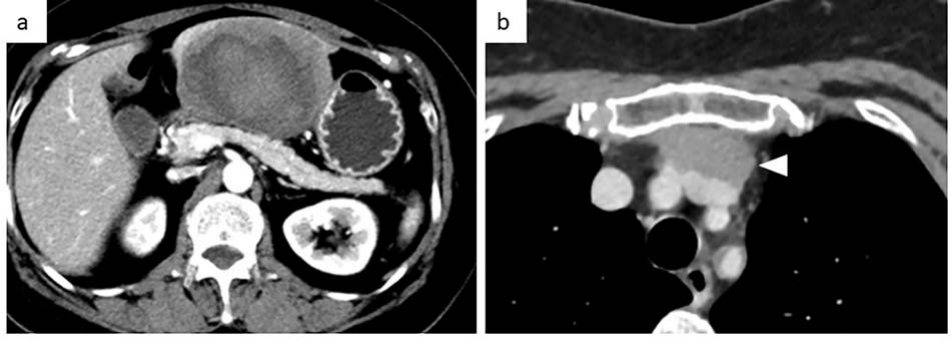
Contrast-enhanced CT. (a) Abdominal CT: a solitary liver tumor, 10 cm in diameter, was located in the left lateral segment of the liver. The tumor had a clear margin, with tumor enhancement in both the arterial and portal phases. (b) Chest CT: an enhanced tumor with an irregular margin was detected in the anterior mediastinum. The tumor had a maximum diameter of 4.5 cm.
